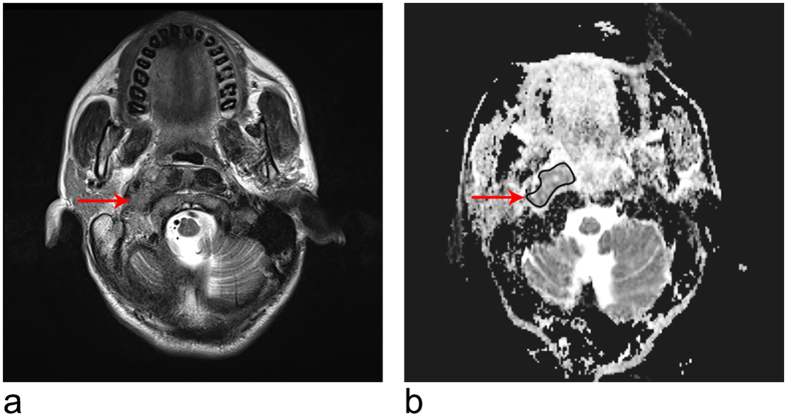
MR images of a 46-year-old woman. (a) Axial T2-weighted fast spin-echo (2470/90) MR image showing the primary tumor (arrow) in the nasopharynx with parapharyngeal extension. The spin-echo echo-planar (5100/96) DW images were obtained with *b* values of 0 and 1000 s/mm^2^. (b) The lesion (arrow) shows intermediate signal intensity on the corresponding ADC map (ADC = 0.893 × 10^−3^ mm^2^/s). The patient experienced local failure 22.8 months after treatment.
